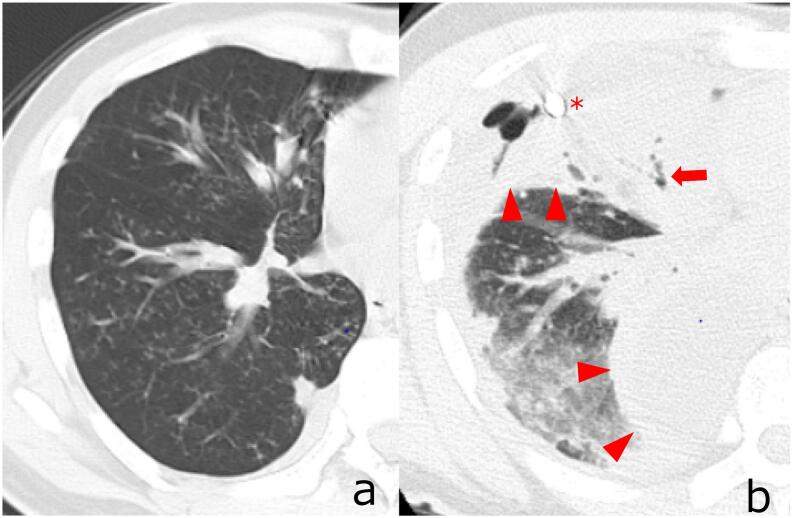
Chest CT before and after admission. a: Chest CT showing diffuse panbronchiolitis 2 weeks before admission. b: Chest CT showing pneumonitis in the right lung. The bronchioles were obstructed by phlegm (arrows). Multifocal pleural effusion is observed in the right thoracic cavity (arrowhead). A chest drainage tube is inserted into the ventral space (asterisk).
